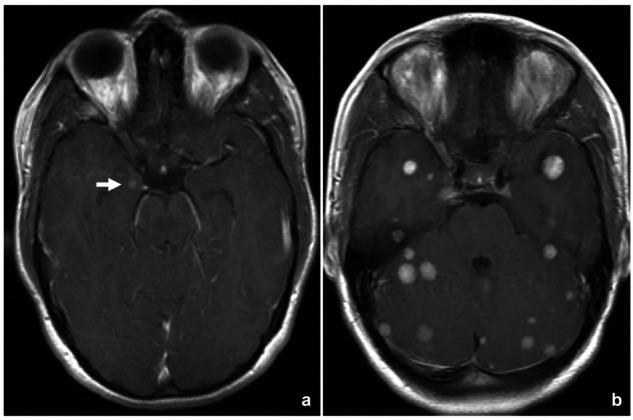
Hyperprogression in a patient with brain metastases from melanoma treated with Ipilimumab without concomitant radiotherapy. Baseline MRI scan 5 days before starting Ipilimumab (a) shows one 4 mm temporal lesion (arrow in a). Follow-up MRI scan 34 days after starting ipilimumab (b) demonstrates a dramatic increase in the number and size of brain metastases, with more than 5 target lesions and more than 15 non-target lesions. The SLD increased to 60 mm, and the patient also experienced a worsening of their neurological status. The rapid progression of tumor burden right after starting treatment is consistent with hyperprogression
